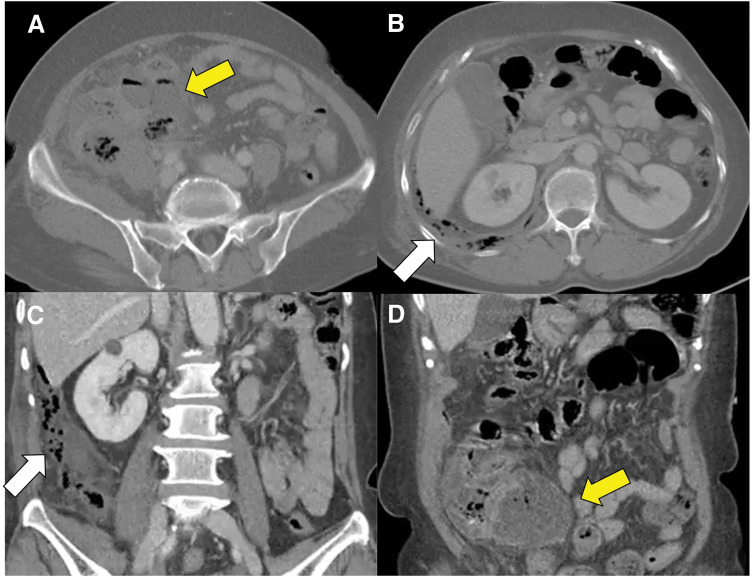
Enhanced CT images show a large 6.0-cm abscess (yellow arrows) in the pericecal area. Mottled air bubbles (white arrows) in the right paracolic gutter indicate pneumoperitoneum (A, B: axial; C, D: coronal).
